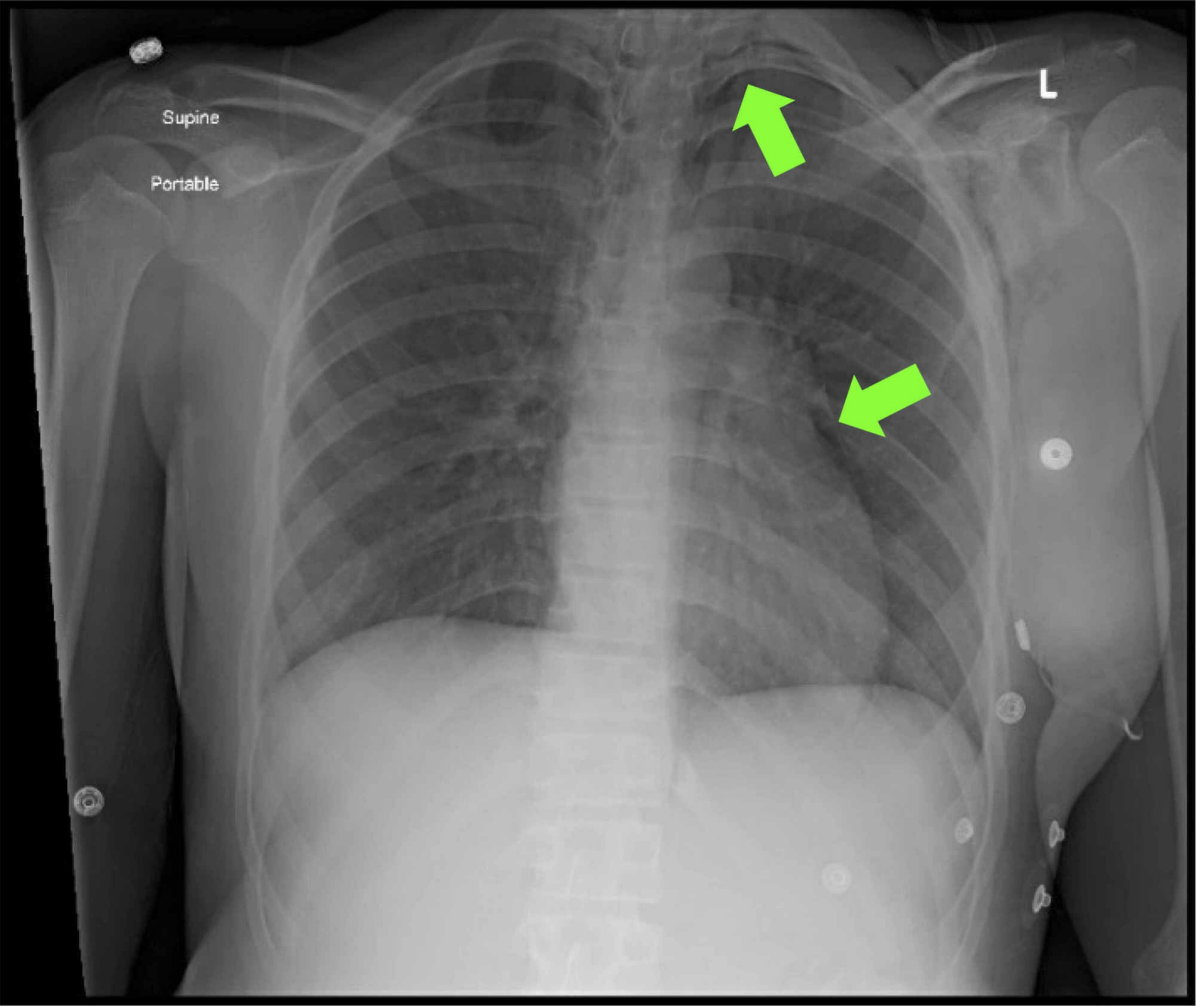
Initial chest X-ray showing pneumothorax and pneumomediastinum
